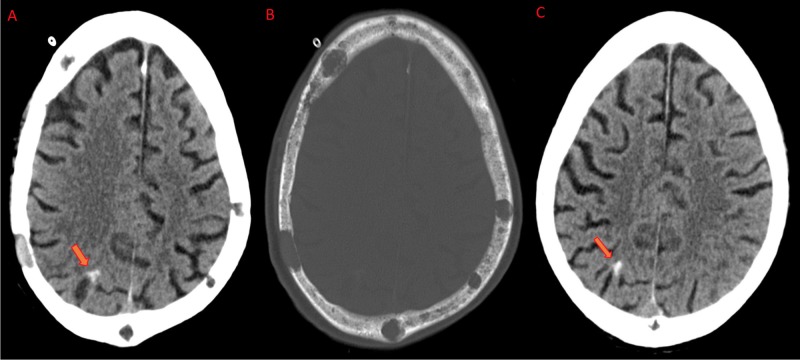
Computerized tomography (CT) axial images A) On the day of admission showing right parietal cortex hyperdense (arrow) lesion with no perilesional edema; B) Skull showing well demarcated, punched out, lytic lesions of multiple myeloma; C) Follow-up CT of brain showed persistent calcification.​
